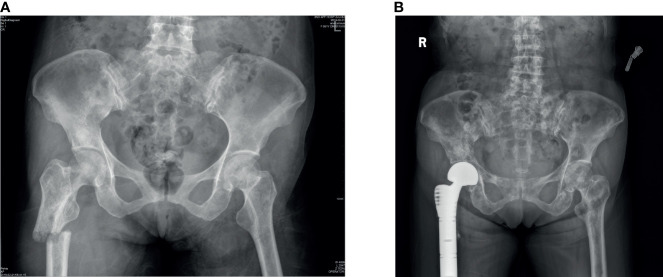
(A) As we can observe from the x-rays that a patient had bone metastases from breast cancer and a pathological fracture of the right femur. (B) The patient underwent right hip replacement surgery.
